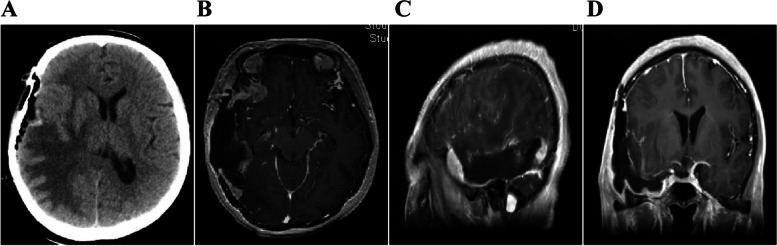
The second craniotomy was performed for the recurrent intracerebral tumor. During the operation, it was found that the reddish-brown supply rich tumor tissue broke through the dura mater and grew deep into the temporal muscle. A Postoperative CT showed that the recurrent tumor was removed, and the surgical field was clean without bleeding. B Enhanced axial view. C Enhanced sagittal view showed that there was still tumor tissue remaining in the frontal lobe. D Enhanced coronal view showed that the recurrent temporal lobe tumor was completely removed
